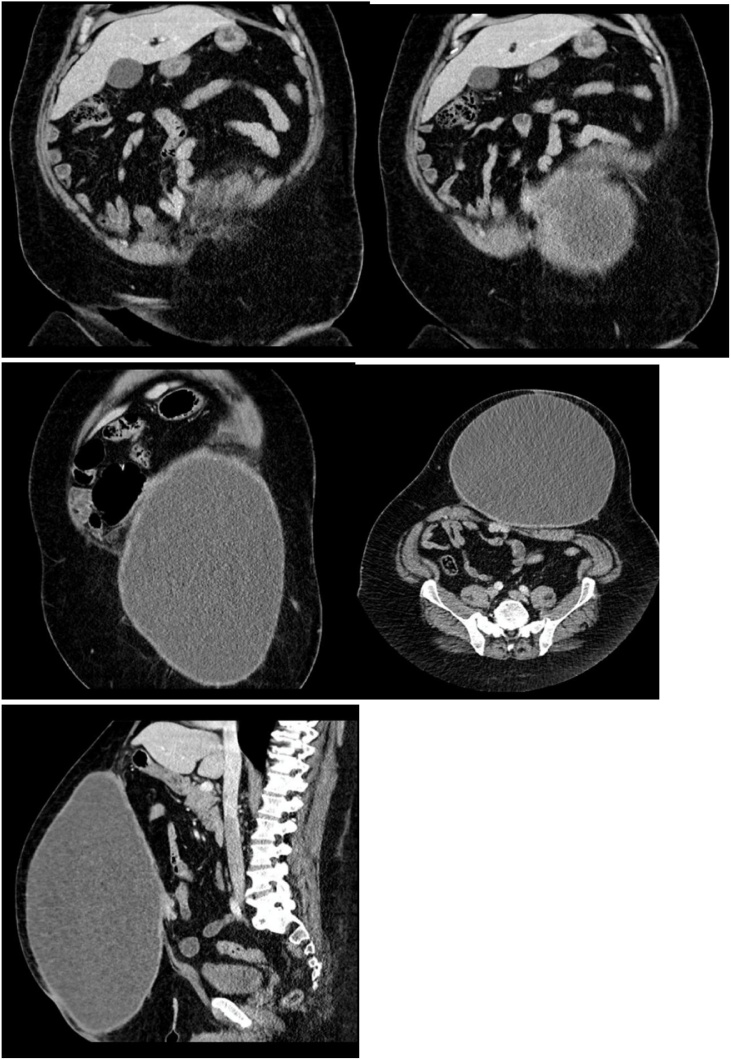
Pre-operative computed tomography imaging demonstrating seroma size.
